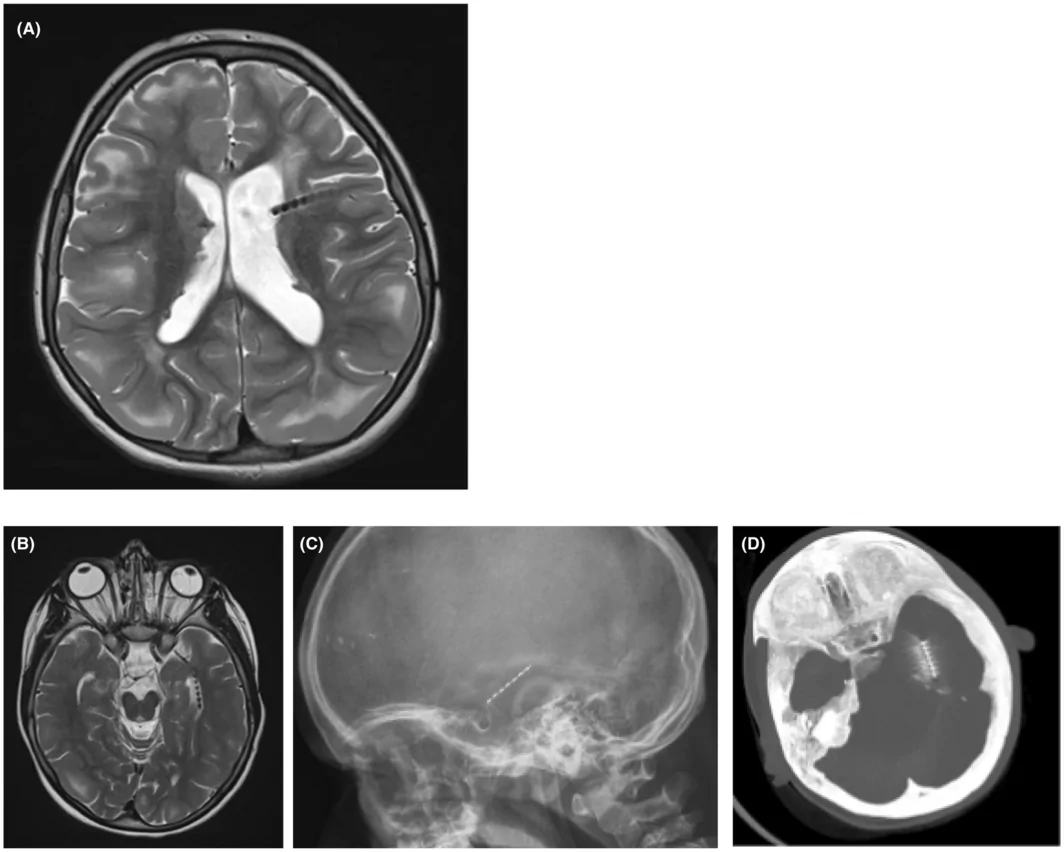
Intracranial electrode fragment migration. (A) Axial brain MRI showing initial migration of the retained electrode fragment toward the left lateral ventricle. (B) Axial brain MRI showing the position of the electrode 1 year later within the left temporal horn of the lateral ventricle. (C) Lateral skull X‐ray showing a linear, radiopaque foreign body adjacent to the left temporal squama. (D) Axial brain CT showing the position of the electrode.
